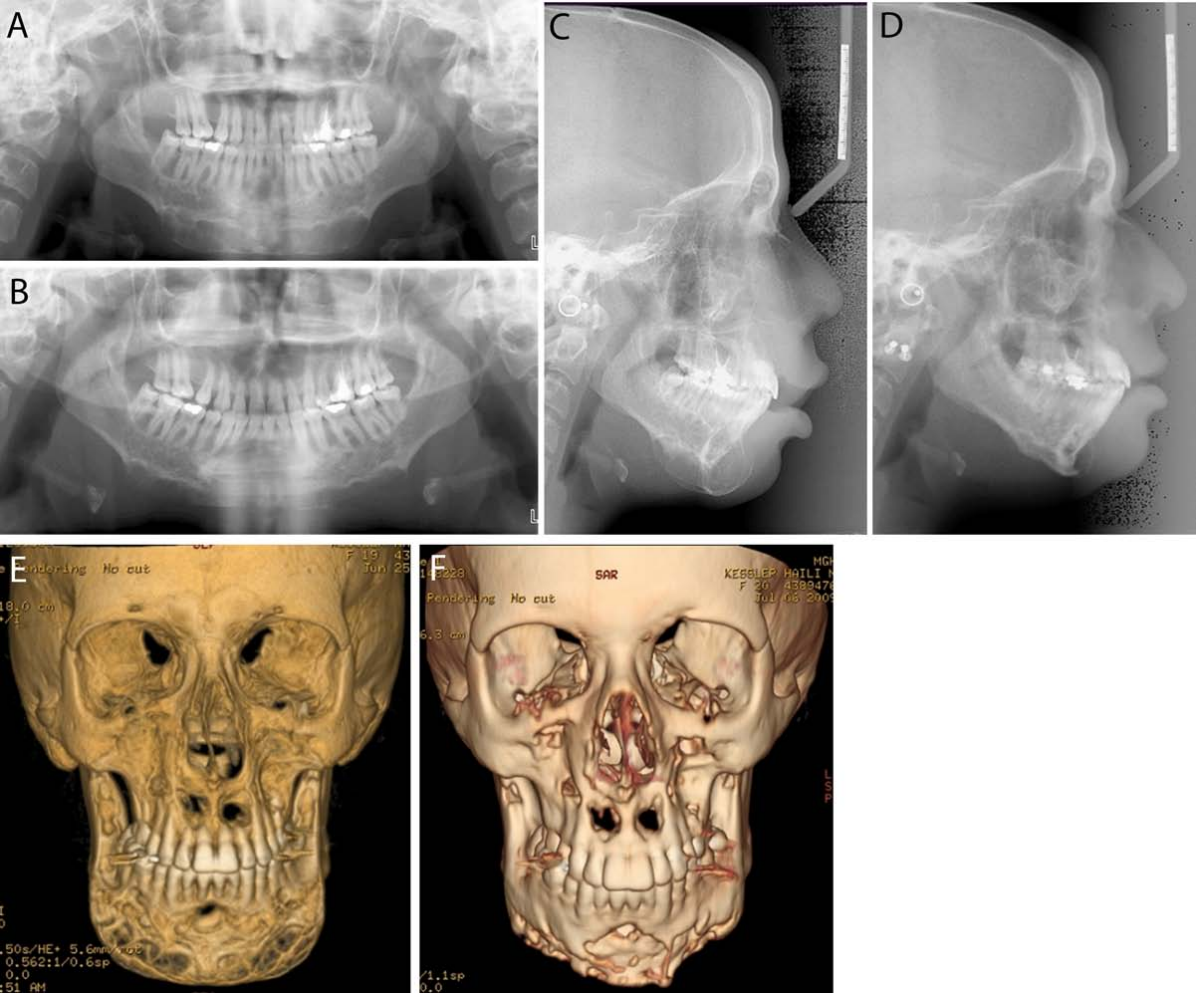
Imaging studies of the patient in Figure 3 pre-treatment (A, C, E) and post-treatment (B, D, F). A and B. Panoramic radiographs. C and D. Lateral cephalograms. E and F. 3D CT scans. Pre- and post-treatment images show improvement of the giant cell lesions and the contours of the mandible.
